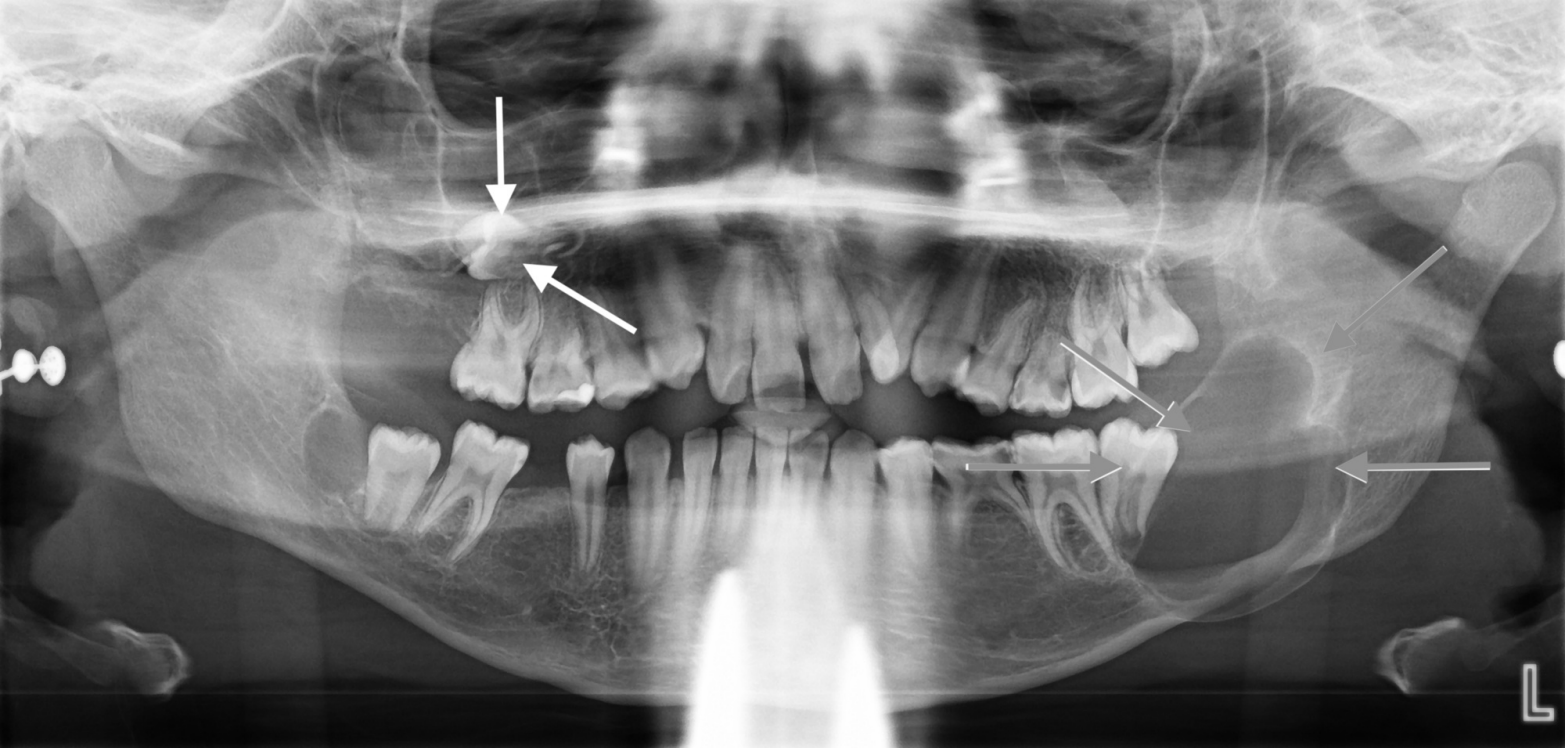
An orthopantomogram (OPG) shows the maxillary and mandibular lesions before the surgery. An orthopantomogram (OPG) shows a lesion associated with a partially erupted mandibular second molar tooth. The left mandibular lesion extended up to the ramus of the mandible (the gray arrows pointing down in the left side); moreover, the patient had another lesion associated with a horizontally impacted second premolar in the maxilla (the white arrows pointing up in the right side).
